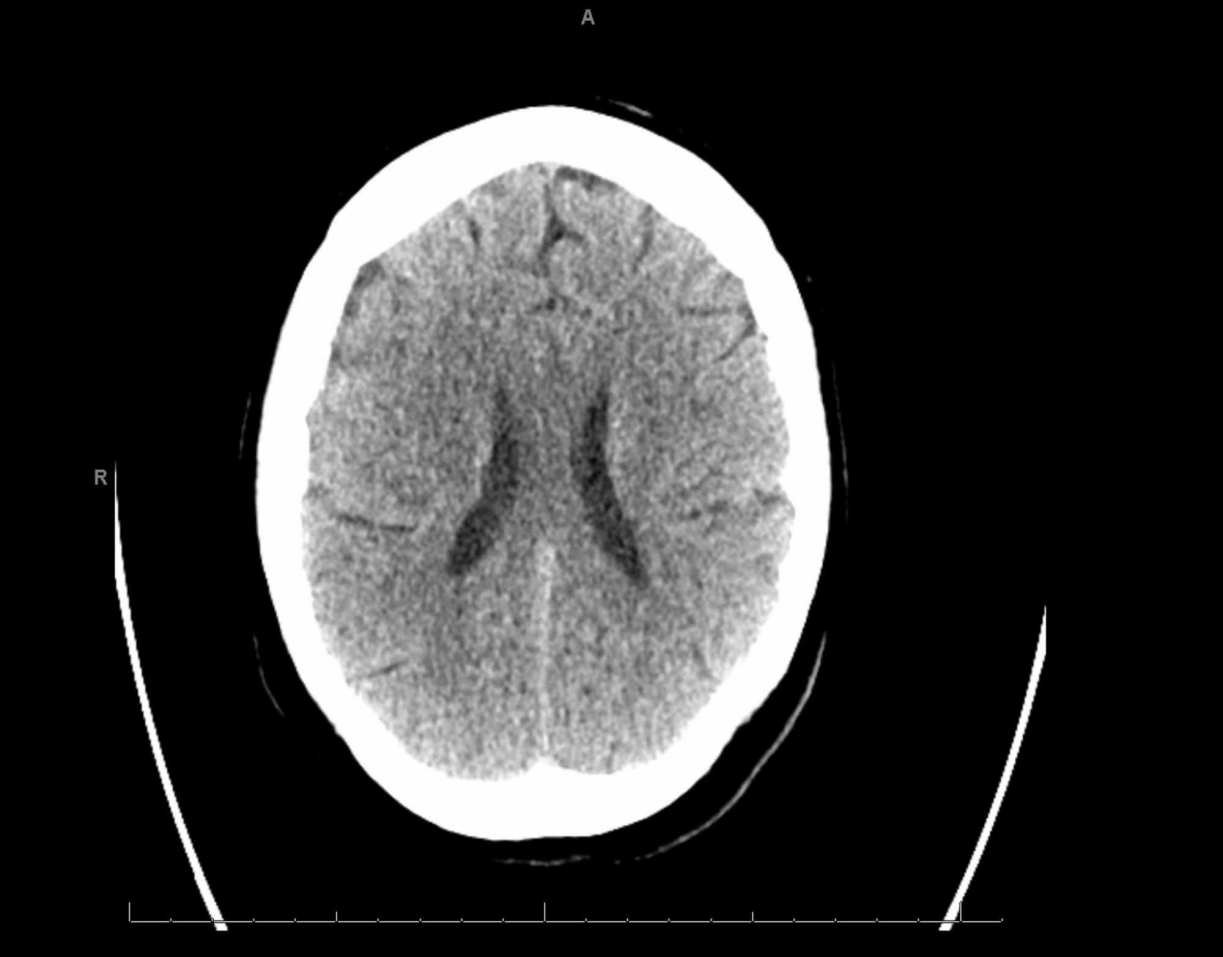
Non-contrast computed tomography (CT) of the head hours after symptom onset without signs of an acute infarct
